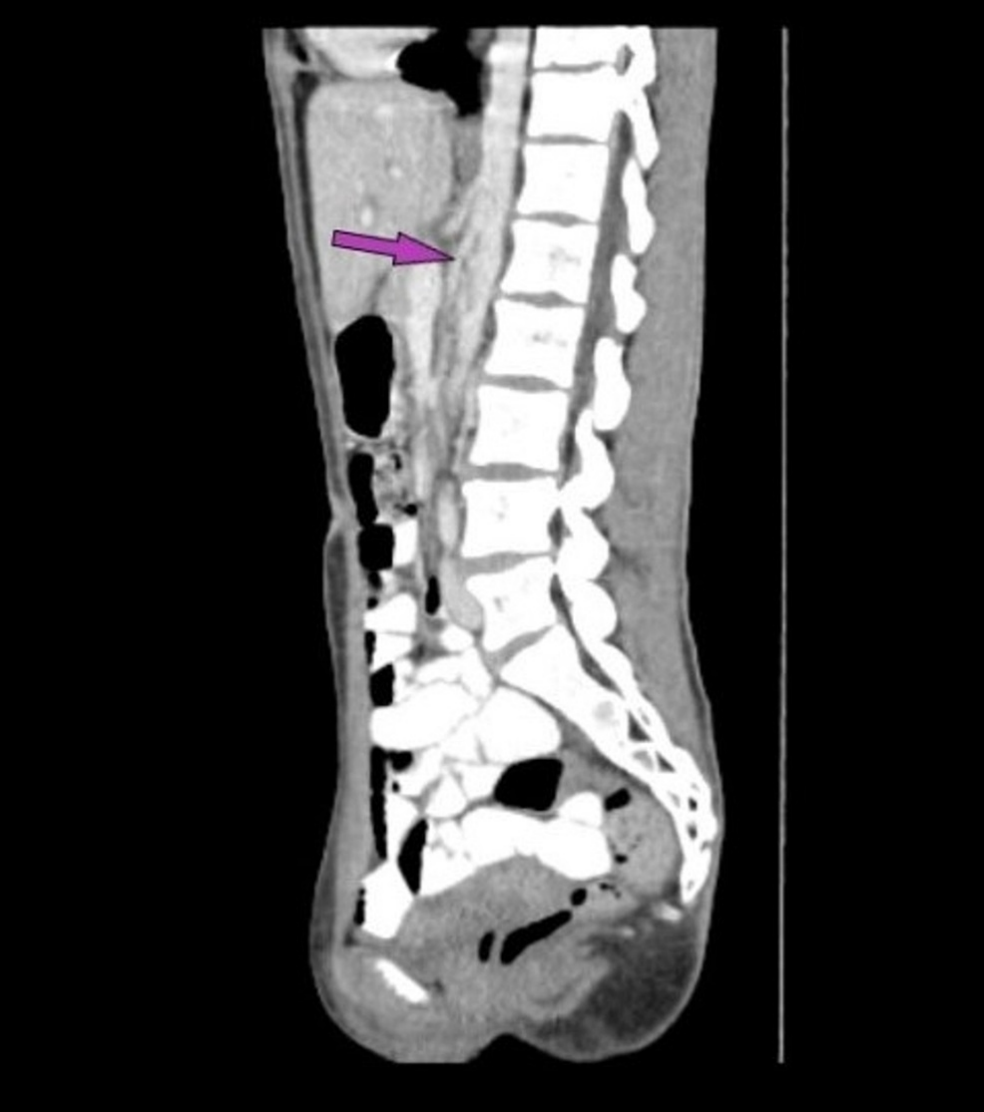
Sagittal contrast-enhanced CT scan demonstrating aortomesenteric angle narrowed to 14 degrees The arrow indicates the superior mesenteric artery.
